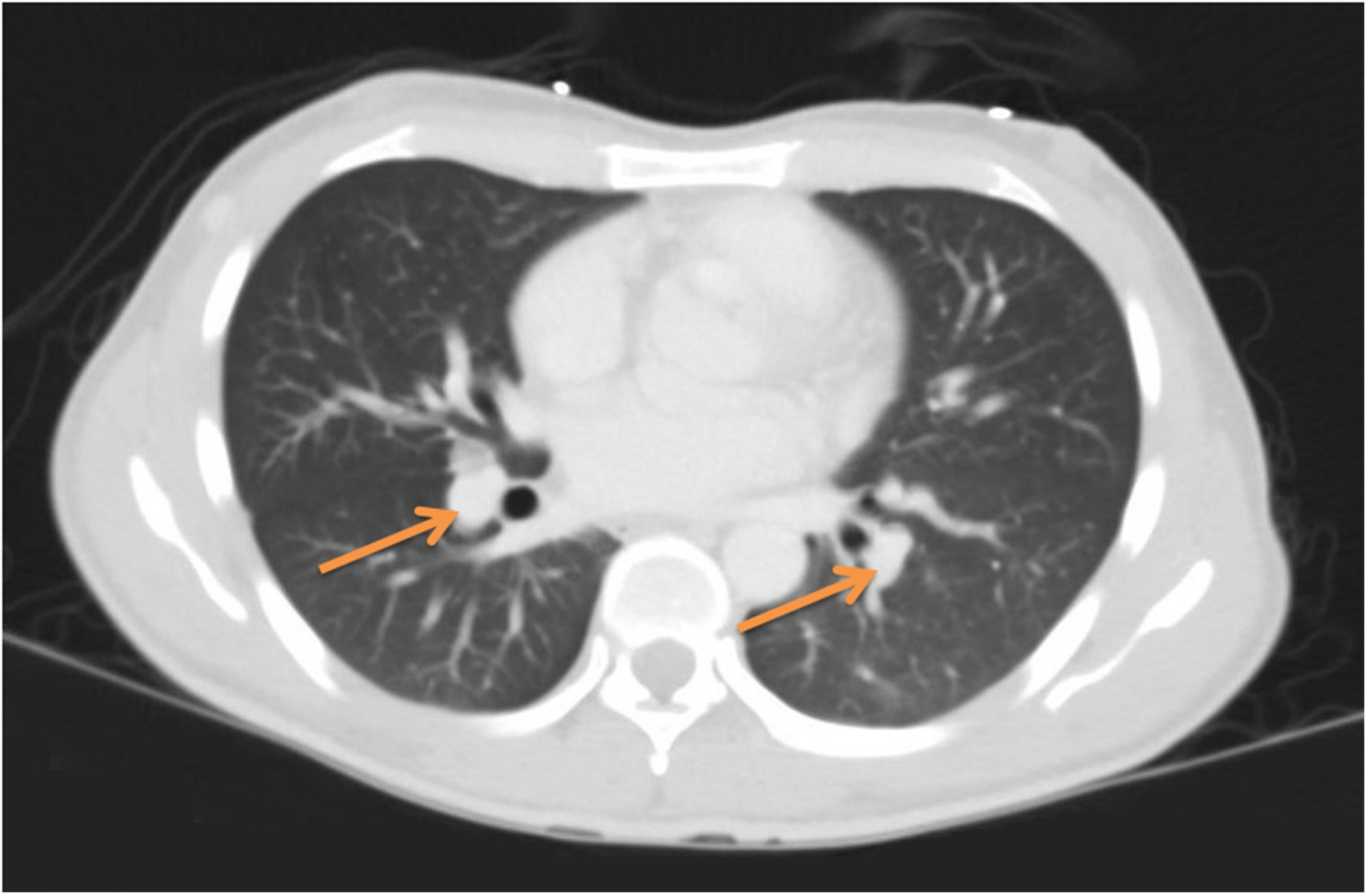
Chest CT scan showing prominent aortopulmonary lymph nodes (arrows).
